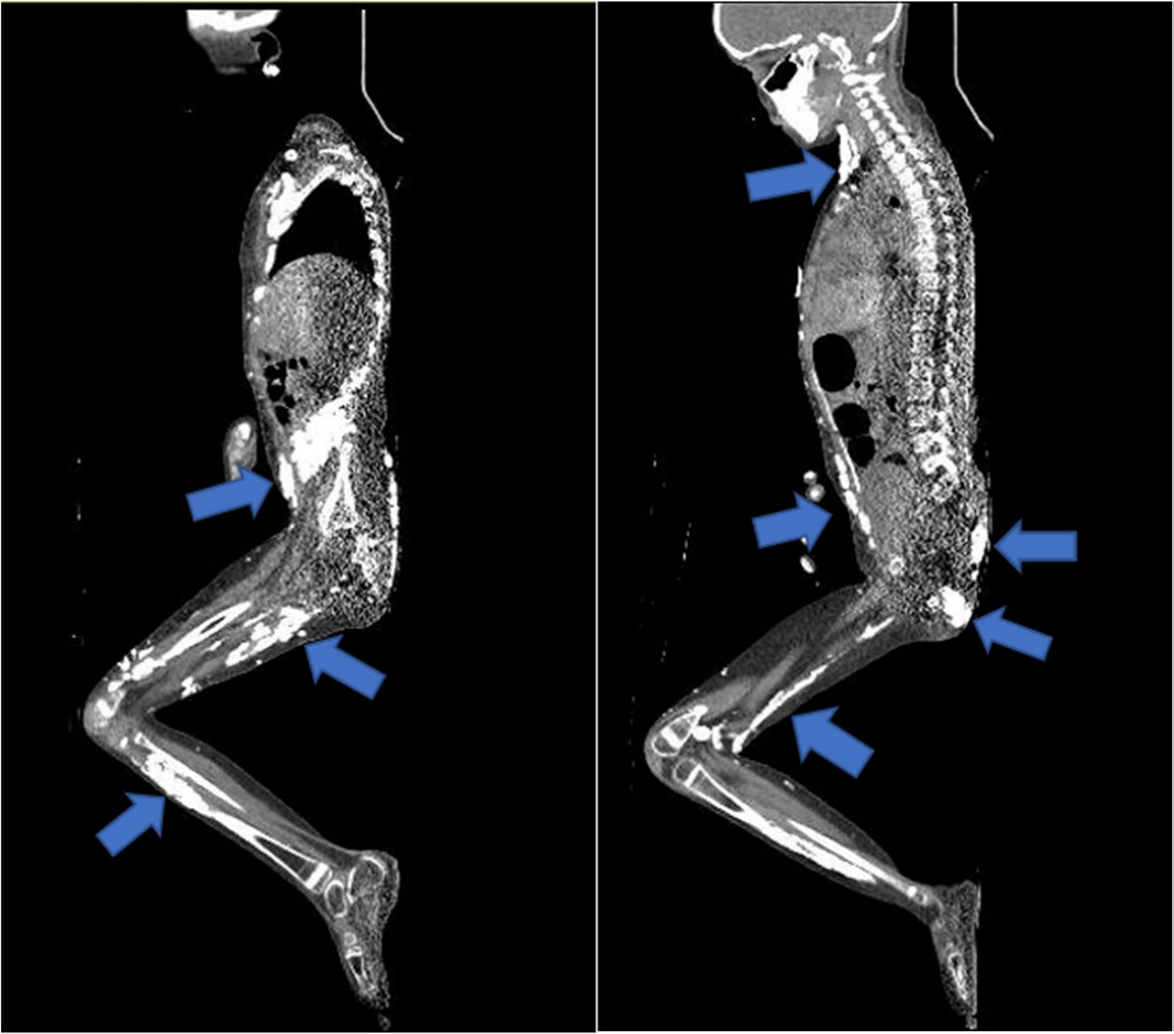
Low-dose whole body CT of a 7-year-old female with refractory JDM and extensive calcinosis universalis. Arrows demonstrate prominent locations of the extensive calcifications in the soft tissues and musculature extending from the neck, along the torso, upper and lower extremities. Also demonstrated are intra-abdominal and pelvic calcifications
